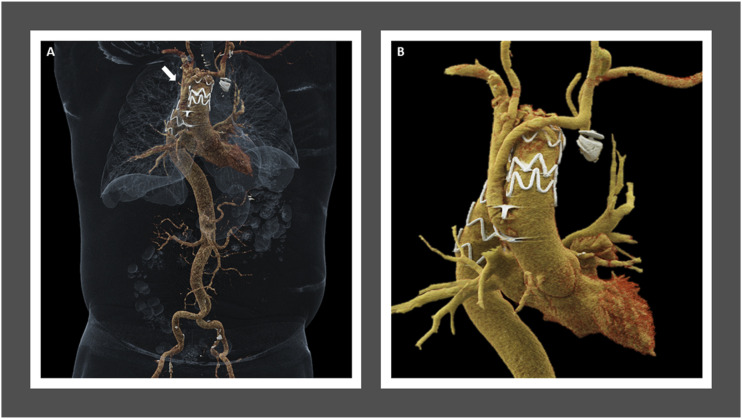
One-year follow-up computed tomography angiogram. Three-dimensional cinematic volume rendering technique anterior thoracoabdominal view (A) ascending and descending thoracic view (B) show right-sided aortic arch and post debranching the great vessels with reimplantation of the proximal aorta, left carotid to subclavian bypass with embolization of the proximal left subclavian artery and endovascular stent graft repair of thoracic aortic aneurysm. Reimplanted great vessels are all widely patent. Endovascular stent graft extends from the mid-ascending aorta to the mid-descending aorta. Redemonstration of type II endoleak (arrow). The distal aortic arch measures 50 mm in the axial plane at the level of the Amplatzer occlusion plug of the left subclavian artery, which is unchanged when remeasured by me at the same level in the prior study.
